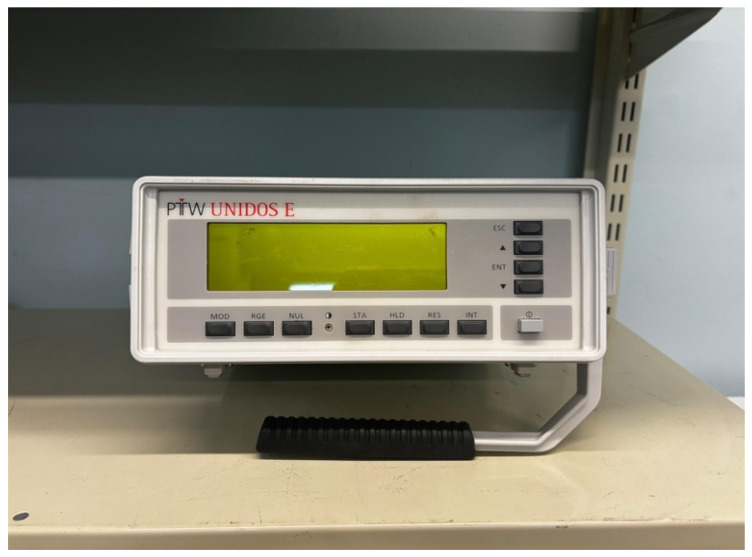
An electrometer to measure the dose output of a CT scanner.
